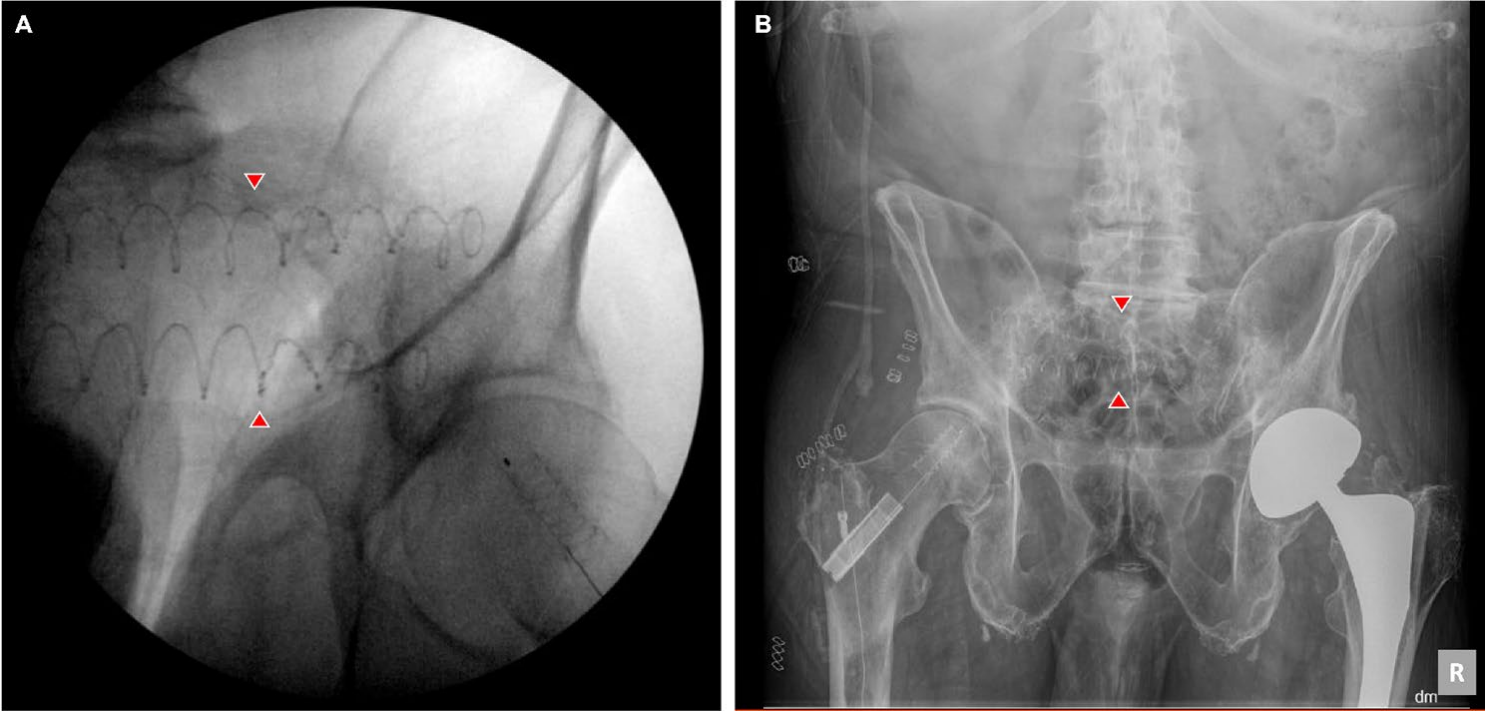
(A) Intraoperative fluoroscopy, and (B) postoperative AP radiograph showing adequate placement of 2 photodynamic nails
